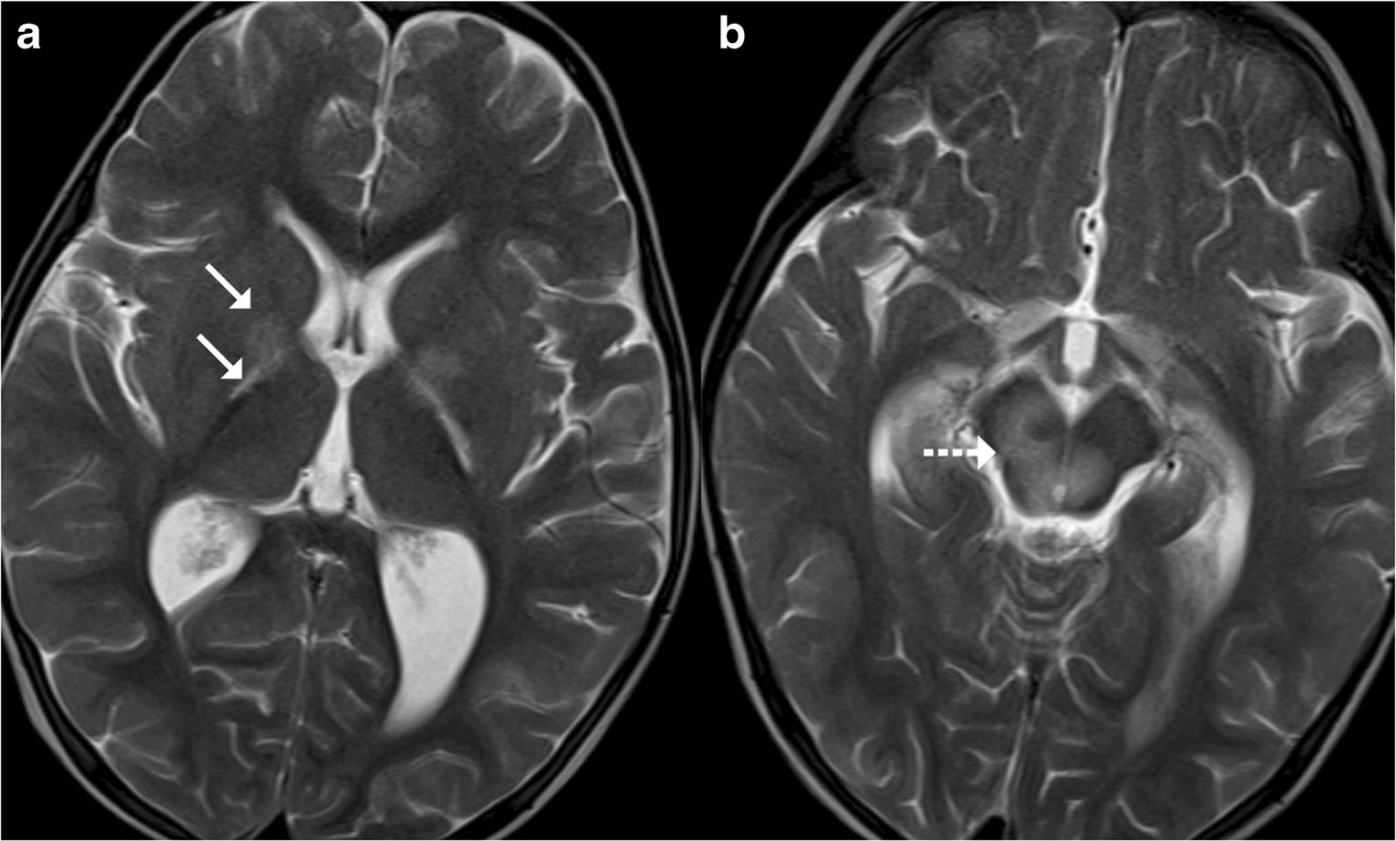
A 6-year-old patient with haemolytic uraemic syndrome and encephalopathy: a–b Axial T2-weighted images reveal increased signal in the basal ganglia, posterior limb of internal capsules (*arrow*) and midbrain (*dotted arrow*)
